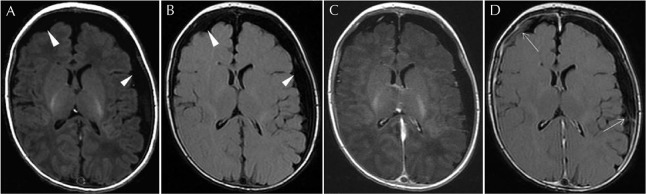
Conventional MRI. (A) T_1_IR and (B) FLAIR show subdural effusion (arrowheads). CE (C) T_1_IR does not show enhancement but (D) FLAIR shows a subtle enhancement in the subdural effusion area (arrows). MRI, magnetic resonance imaging; FLAIR, fluid attenuated inversion recovery; T_1_IR, T_1_ weighted inversion recovery.
